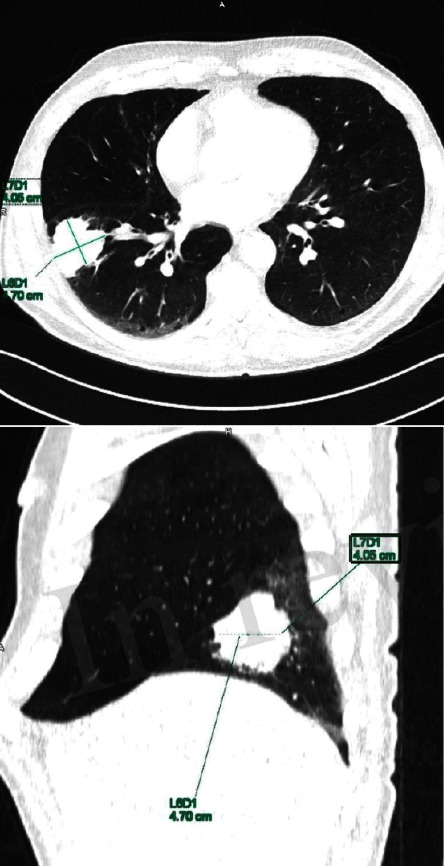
CT of thoracic organs showing an expansive formation with infiltration of the costal pleura in the lower right lung lobe accompanied with a nodular formation which seemed to most likely correspond to metastasis.
